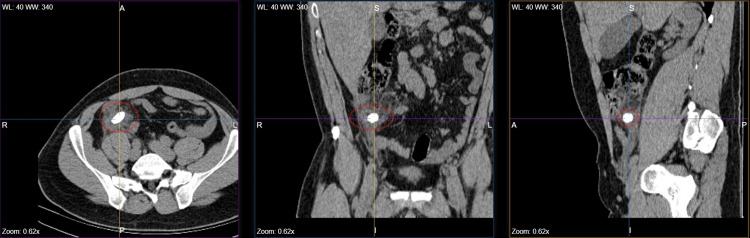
CT scan revealed a giant appendicolith, its exact size and location, and radiological signs of appendicitis.
